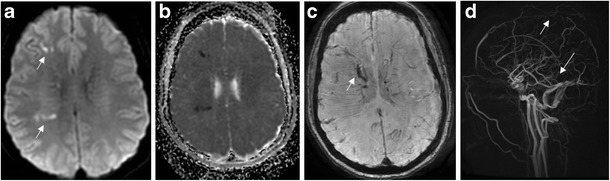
Venous infarct. Forty-three-year-old woman with headache and increased somnolence. DWI (a) and ADC (b) shows punctate foci of reduced diffusion in the right centrum semiovale (*arrows*). Susceptibility-weighted imaging (SWI) (c) demonstrates linear susceptibility along callososeptal veins, right greater than left (*arrow*). Magnetic resonance venography (MRV) (d) demonstrates loss of flow-related enhancement in the superior sagittal sinus and expected location of the internal cerebral veins, vein of Galen, and straight sinus (*arrows*)
